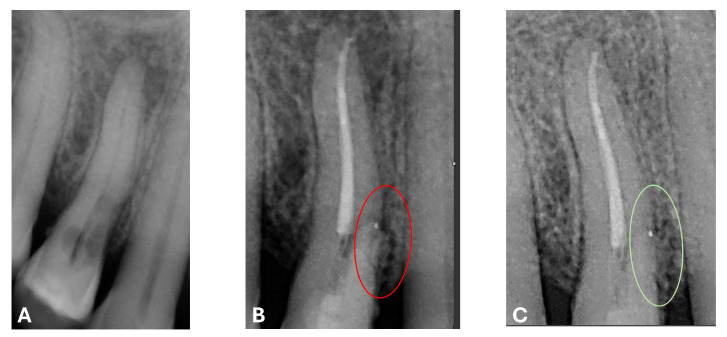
EPL before (A) and after treatment (red circle showing mesiocervical widening of the lamina dura space) (B) and at 12-month follow-up (C) with radiographic evidence of restoration of the lamina dura (green circle), periodontal ligament space and absence of PL.
